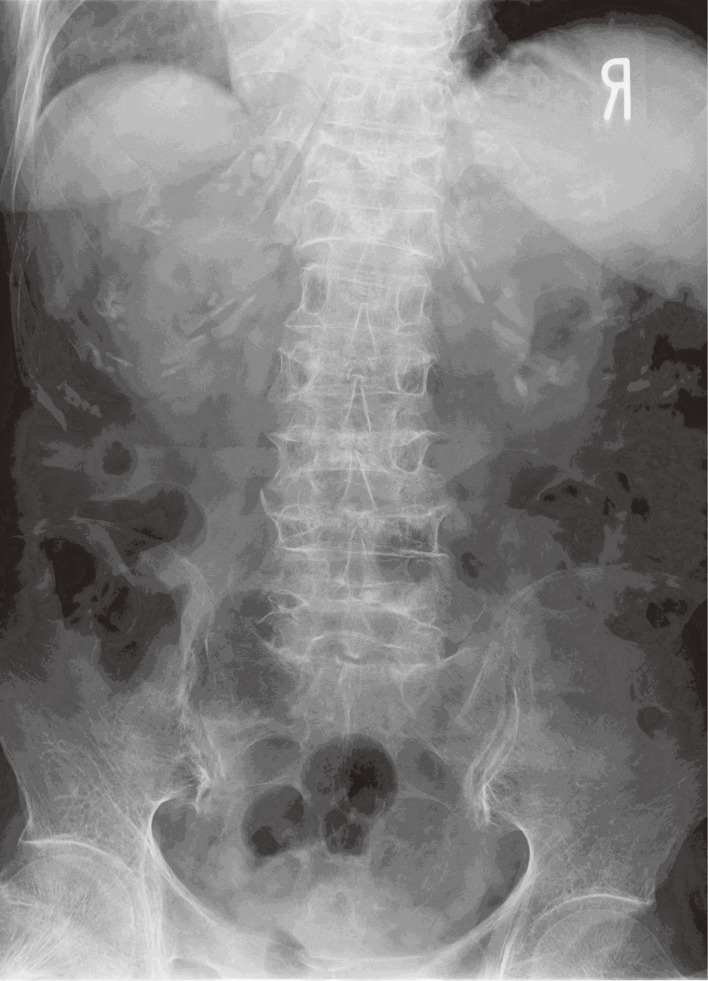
An X-ray photograph of thoracic and lumbar vertebrae from the front.
